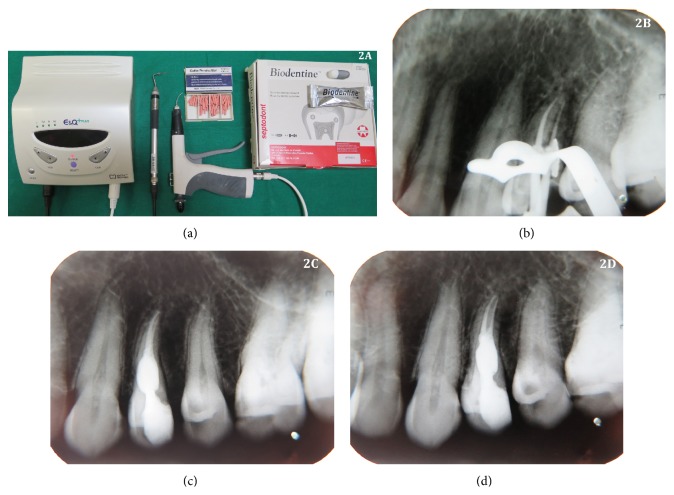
(a) E & Q (META BIOMED) system apparatus (pen, gun, and gutta-percha bar) and Biodentine capsule (Septodont, Saint-Maur-des-Fossés, France). (b) Confirmatory radiograph after placement of Biodentine. (c) Immediate postoperative radiograph. (d) Ten-month follow-up radiograph.
